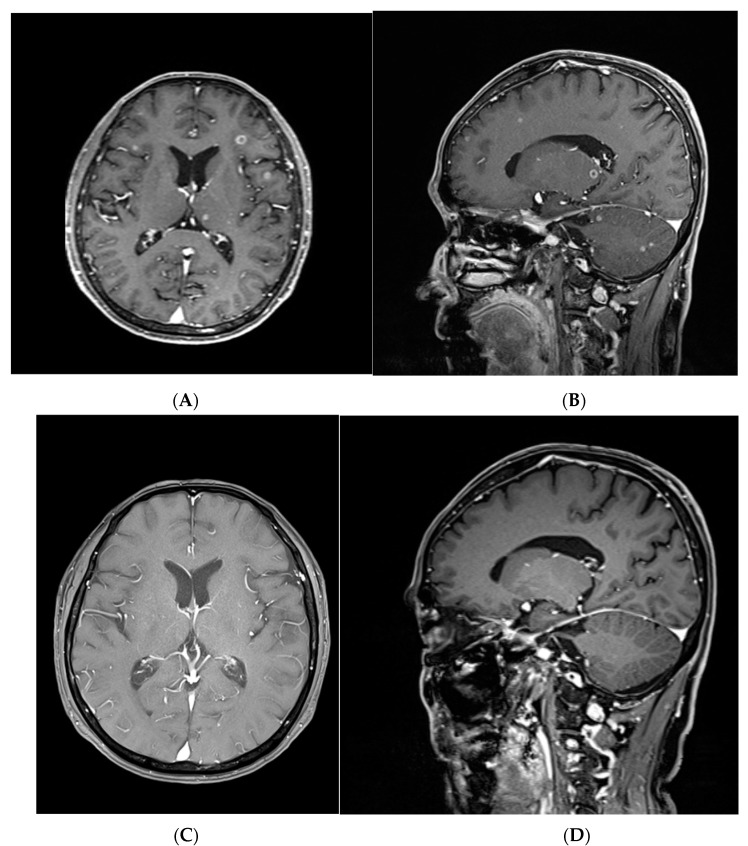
A representative case treated with targeted therapy without local brain treatment. (A): Multiple heterogeneously enhancing masses (maximum 0.8 cm-sized) in both the cerebral hemispheres with perilesional edema on axial T1-weighted MRI with gadolinium enhancement. (B): Multiple heterogeneously enhancing masses (maximum 0.2 cm-sized) in both the supratentorial and infratentorial lesions with perilesional edema on sagittal T1-weighted MRI with gadolinium enhancement. (C): The axial brain T1-weighted MRI with gadolinium enhancement showed complete resolution five months after afatinib treatment. (D): The sagittal brain T1-weighted MRI with gadolinium enhancement revealed complete resolution five months after afatinib treatment.
